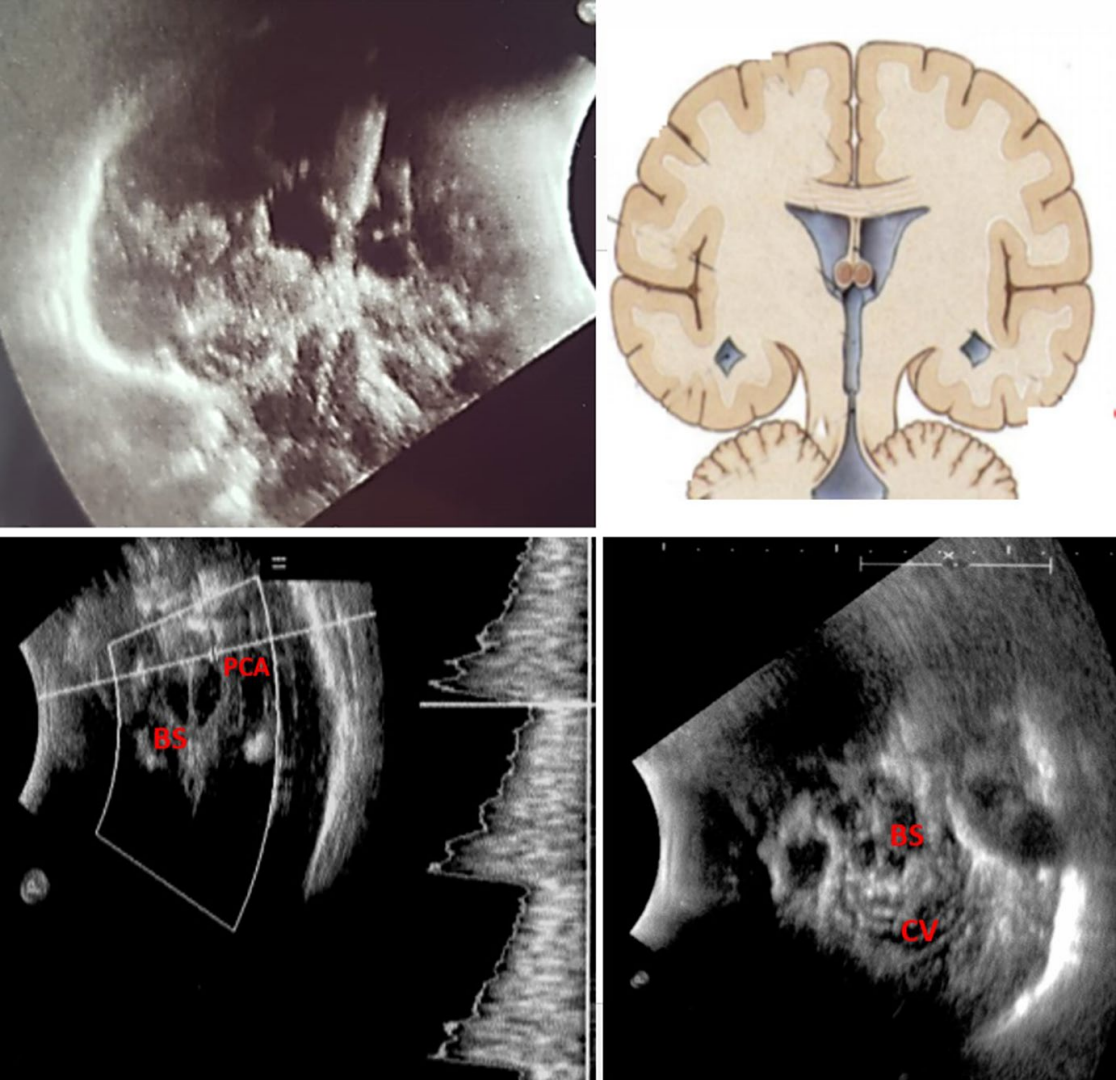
Exemplificative picture showing the possibility to study the whole intracranial anatomy through fronto-temporo-parietal PEEK cranioplasty. Upper row: the TCS probe is placed in a “coronal” projection; bran parenchyma, lateral ventricles, third ventricle, interhemispheric fissure, tentorium, mesencephalon and brainstem are clearly visible and easily recognizable (a similar anatomic projection in on the right as reference). Lower row: through the fronto-temporo-pariental window, posterior cranial fossa anatomy can be easily studied; Doppler and color-doppler tools can, indeed, be used to assess perfusion. BS, brainstem, CV, cerebellar vermis, PCA, posterior cerebral artery, PEEK, polyetheretherketone, TCS, transcranial sonography
